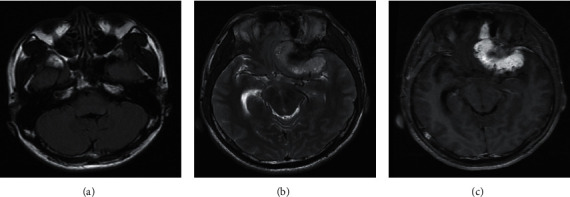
MRI image of a patient with fibrous meningioma (male, 62 years old, left temporal tumor). (a) The patient shows high and low confounding signals on the axial T2WI, with low signal at the edge. (b) Low signal on the axial T1WI with uneven signals. (c) Axial T1WI shows that the edge of the tumor is unevenly enhanced.
